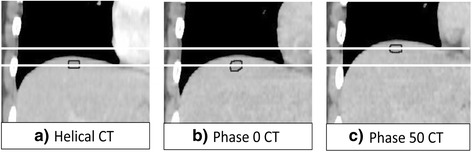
An example of adaptive deformation from (a) helical CT to (b) phase 0 CT and (c) phase 50 CT. The amplitude of liver motion in the SI direction is shown as the distance between the two white lines
